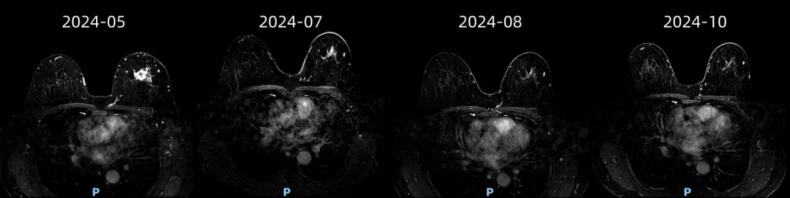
After the start of neoadjuvant therapy, breast MRI should be rechecked every two cycles, and the curative effect is partially relieved.
